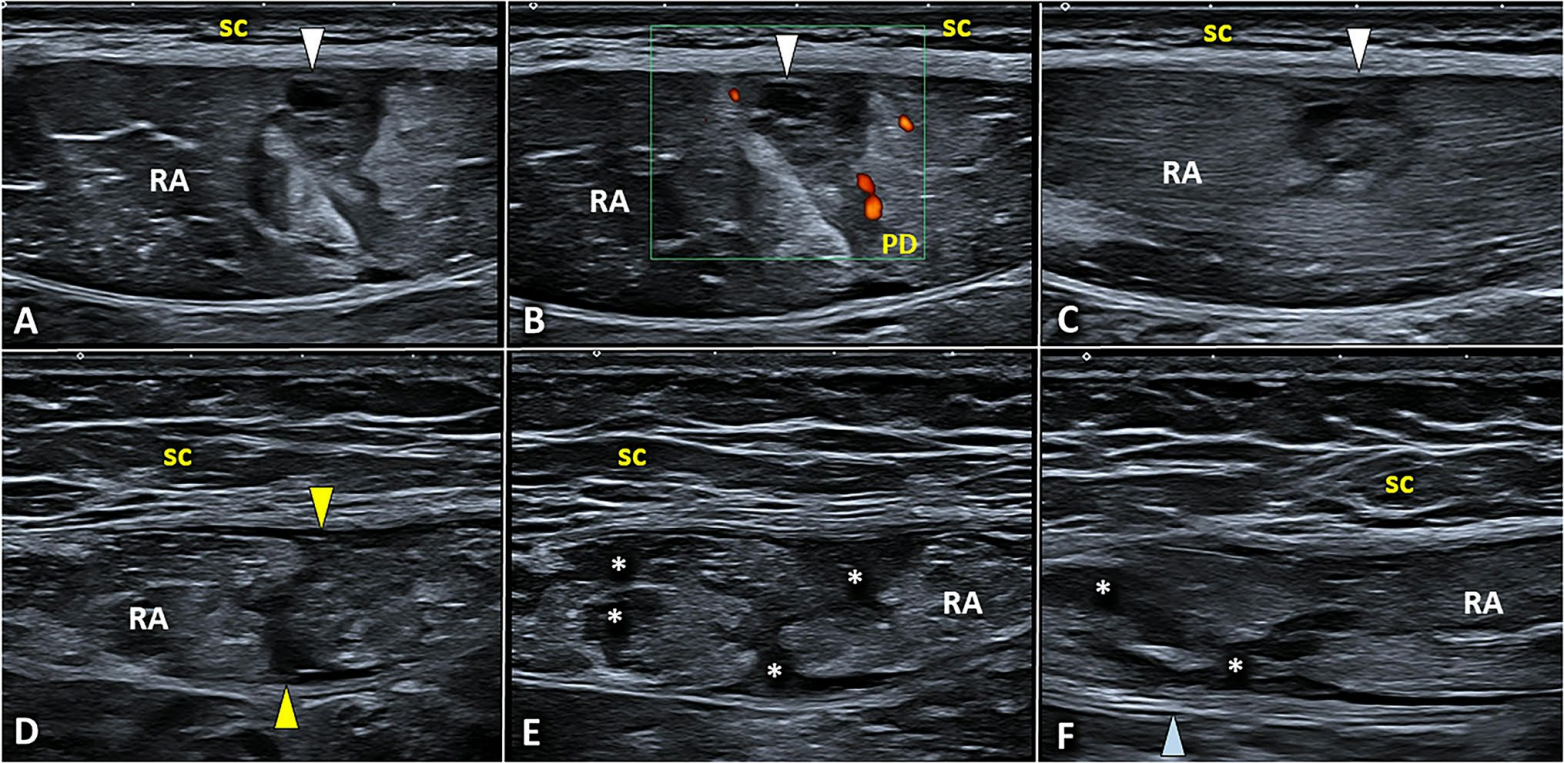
Combining transverse (A, B) and longitudinal (C) scans, a focal injury *(white arrowhead)* involving the superficial fibers of rectus abdominis muscle *(RA)*—with a mild peri-lesional hypervascularization (B) in power Doppler *(PD)*—can be observed in a professional padel player due to a powerful overhead stroke with massive contraction of the core muscles. Instead, a full-thickness tear *(yellow arrowheads)* of the rectus abdominis *(RA)* with blood collections *(white asterisks)* within the muscle belly can be visualized in transverse sonograms of an amatorial padel player (D, E). Of note, the longitudinal scan (F) confirms the anatomical integrity of the deep lamina of its fascial sheath *(blue arrowhead)*. *sc* subcutaneous tissue
